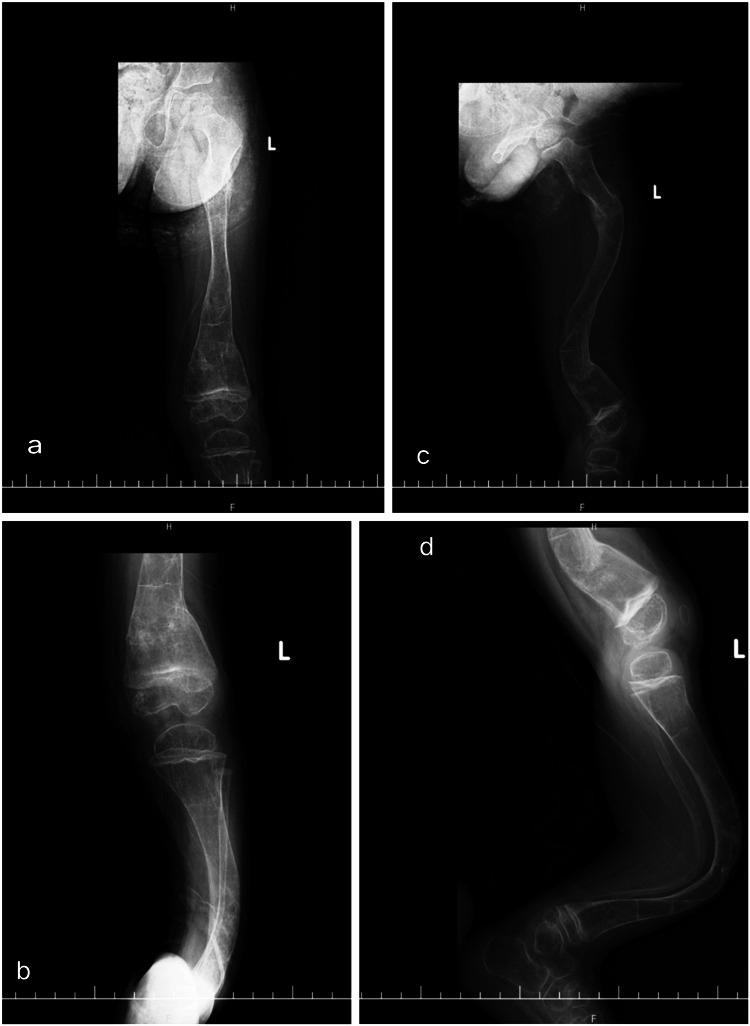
(a-d) Plain radiographs (anteroposterior and lateral views) showing deformity of the left lower limb
